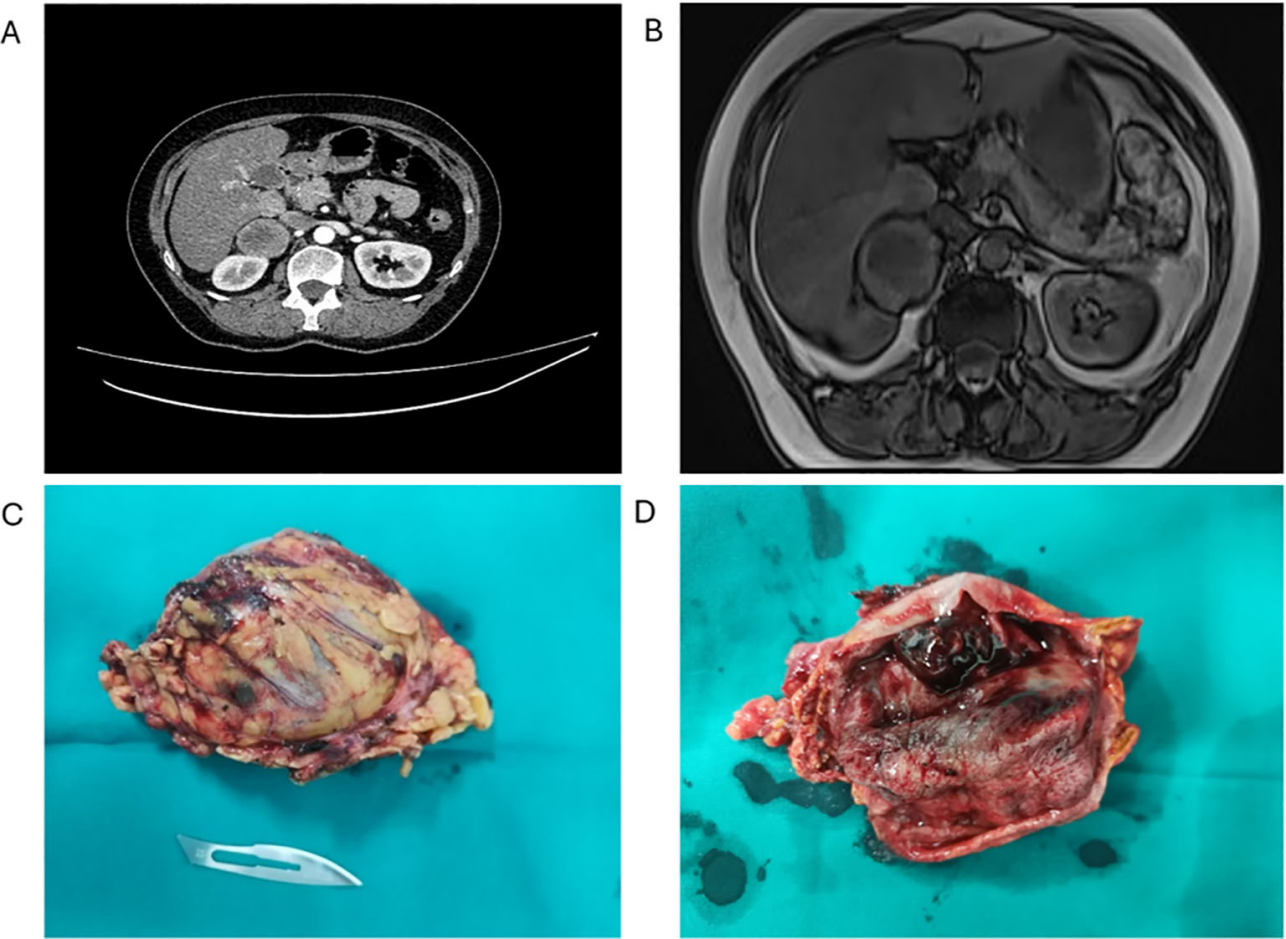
Preoperative CT scan and macroscopic appearance of the tumour. (A) Abdominal CT showing an expansive solid cystic-necrotic mass (arrow) in the cavity of the right adrenal gland, 5.5 x 5.7 cm in size, with continuity in the right lobe of the liver without infiltration, posteriorly pushing into the kidney. (B) Abdominal MRI showing a cystic mass indicative of a necrotic tumour, having a wall of irregular thickness, pushing into the right lobe of the liver with signs of possible infiltration (arrow). (C) Macroscopic appearance of the ACT (81 X 49 x 38 mm) after adrenalectomy. (D) The tumour was well-defined, round and encapsulated, with characteristic brown cross-section.
